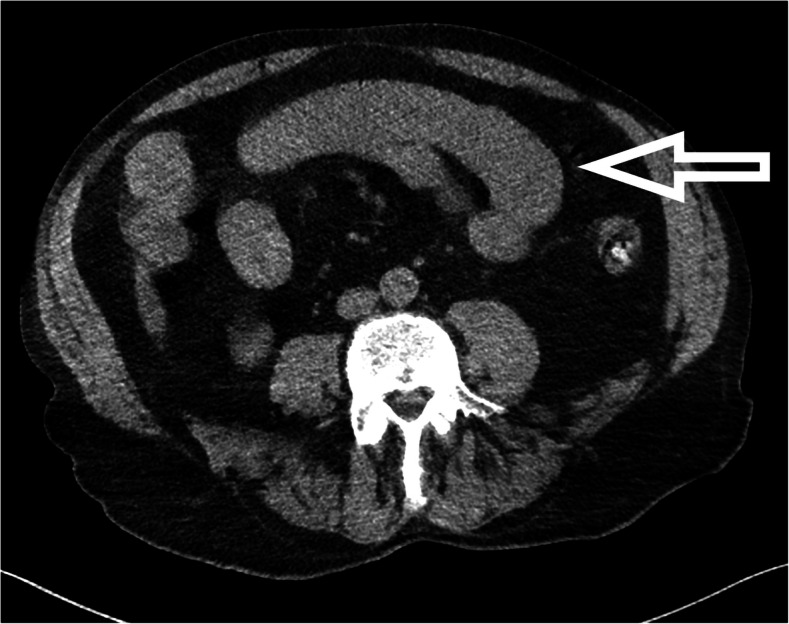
Abdominal CT-scan image of the patient on day 1. Native spiral-CT image of thorax, abdomen, and pelvis with soft tissue window and a 3-mm slice width in the axial plane. The thickened jejunal wall can be seen on the reconstructed image (marked with an arrow)
